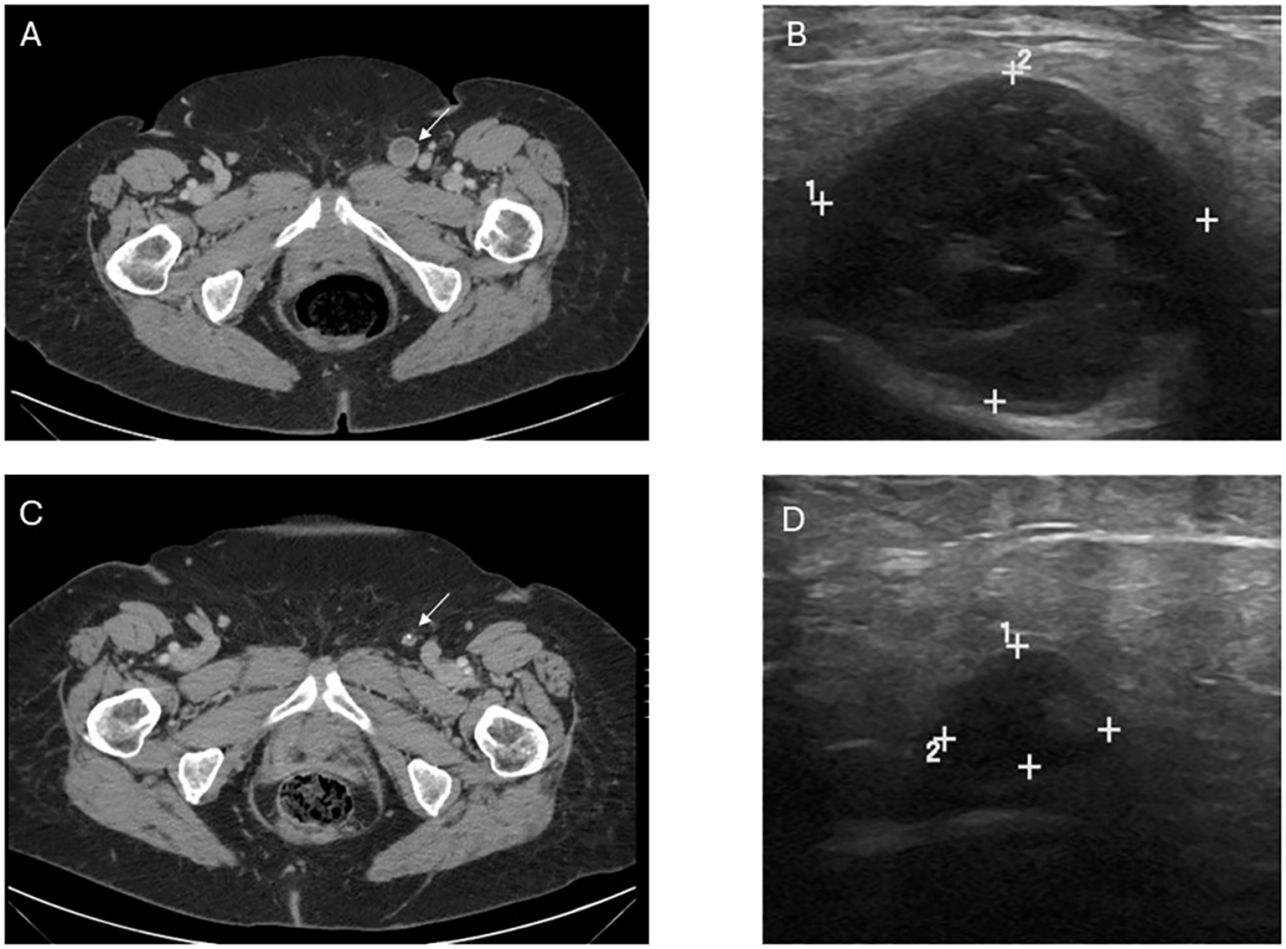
Image findings before and after T-VEC treatment. (A) Computed tomography (CT) demonstrating the abnormal lymph node consistent with metastatic melanoma prior to treatment. (B) Pretreatment ultrasound showing the enlarged, hypoechoic lymph node in the left inguinal region corresponding with the CT finding and measuring 2.3 x 2.1 x 2.7 cm. (C) Post-treatment CT demonstrating decrease in size and near resolution of lymph node, in which fine needle aspiration was unable to retrieve any viable melanoma. (D) Post-treatment US with significant decrease in size, measuring 1.2 x 0.5 x 0.7 cm though with some persistent abnormal morphology. Post-treatment size and morphology remained stable on axial and sonographic imaging after treatment discontinuation.
